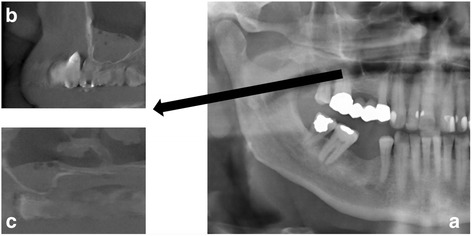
a Panoramic radiography with area of interest (maxillary sinus) and b, c examples of corresponding images in cone beam computed tomography
